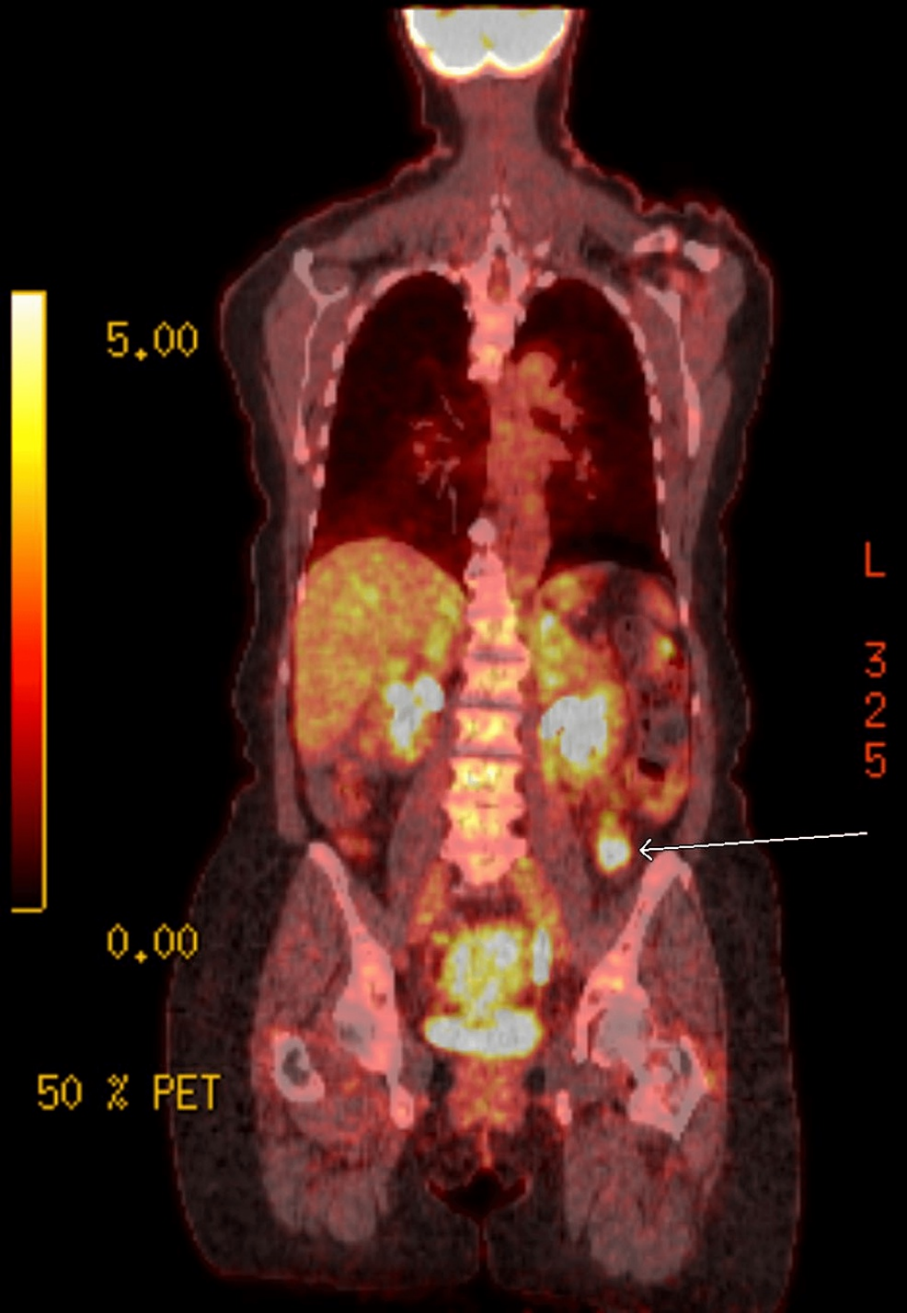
Coronal view positron emission tomography/computed tomography of the abdomen showing hypermetabolic activity in the distal colon (white arrow).
